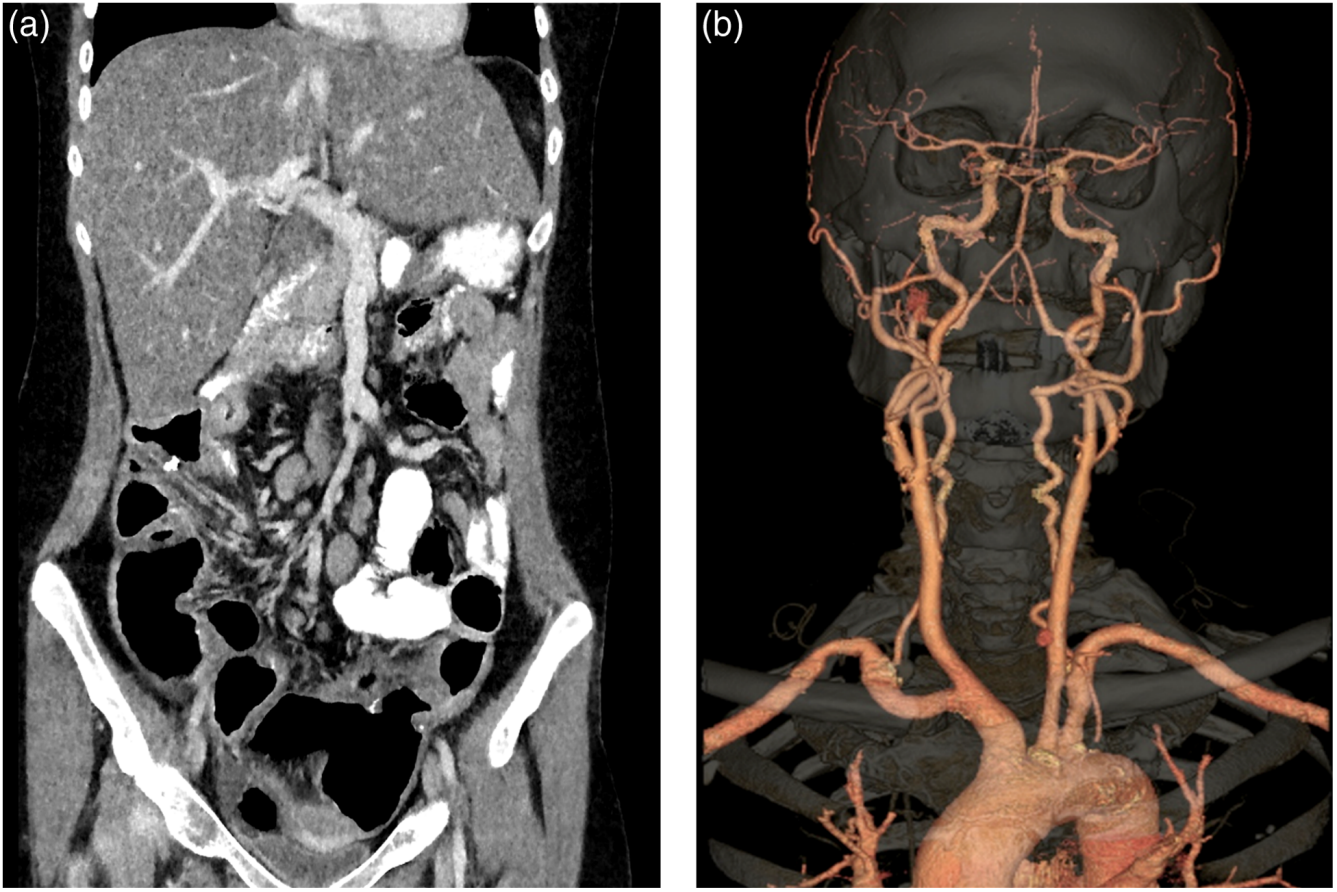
Clinical images acquired on a 64-slice scanner (a) coronal image of an abdomen and pelvis study and (b) volume rendered image of a carotid with circle of Willis CTA study.
